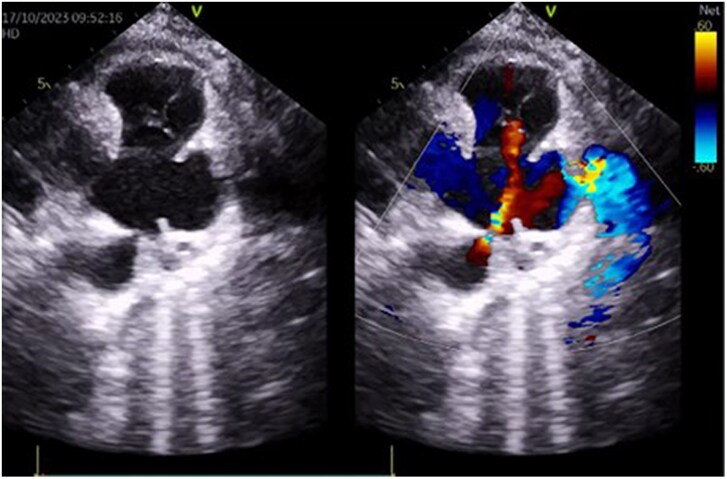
Transthoracic echocardiogram showing a patent ductus arteriosus with a ruptured large aneurysm on the trunk of the pulmonary artery.
